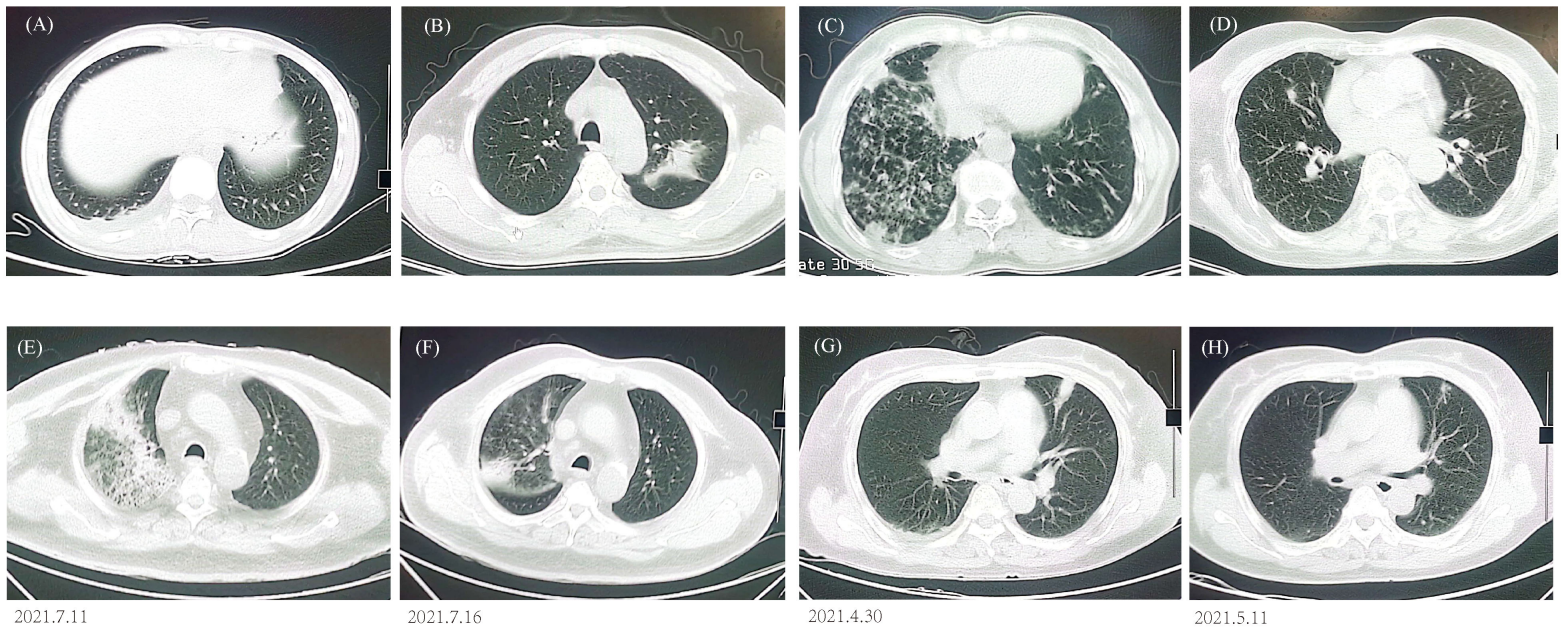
Chest CT findings. (A)
